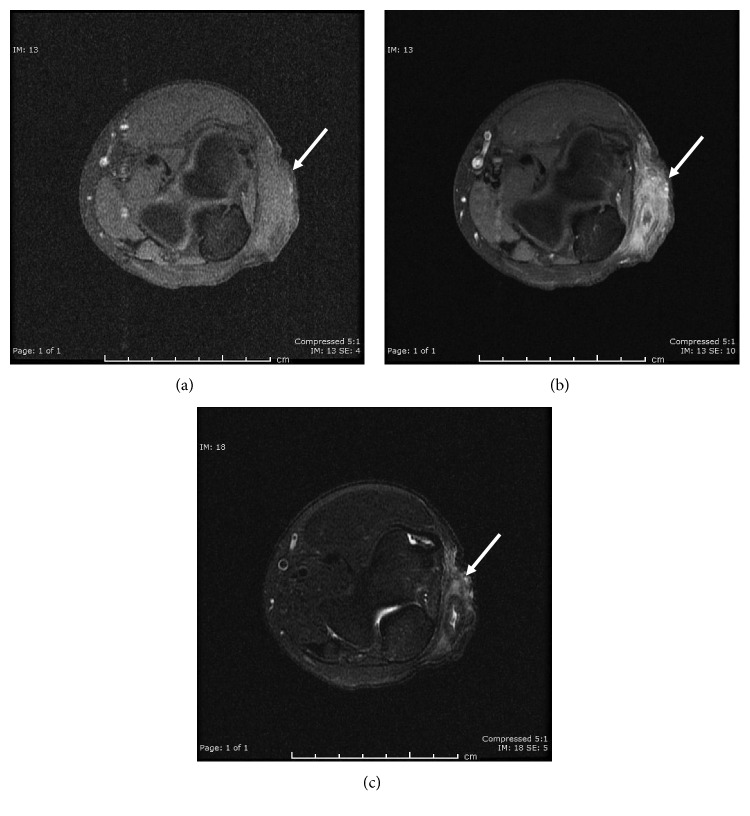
Pre- and postcontrast magnetic resonance imaging of right olecranon. (a) Axial T1 fat-saturated image demonstrates nonspecific T1 isointense mass-like enlargement of the subcutaneous soft tissues overlying the anconeus muscle along the posterolateral aspect of the elbow. (b) Axial T1 fat-saturated postcontrast image demonstrates central hypoenhancement correlating with T2 hyperintense phlegmonous collection (c). There is intense surrounding enhancement representing inflammation. (c) Axial T2 fat-saturated image demonstrates a 1.1 × 2.3 cm T2 centrally hyperintense, peripherally hypointense, phlegmonous collection with diffuse surrounding T2 hyperintense signal representing edema. The phlegmonous collection extended approximately 8 cm in largest dimension.
